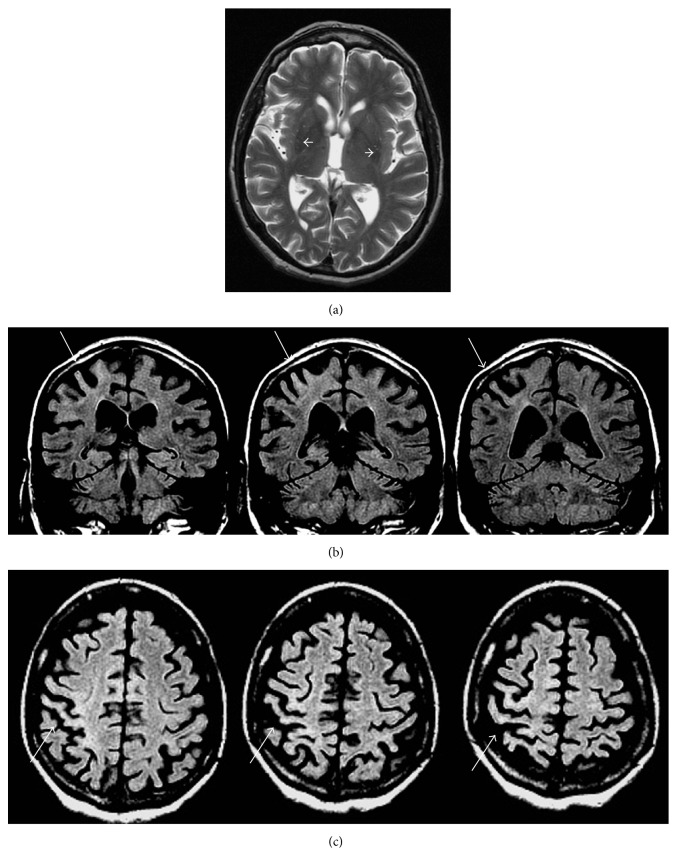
MRI changes disclosed in CBS. (a) Bilateral posterior putaminal T2-hypointensity (white arrows), mainly in the right side, where the putamen appears atrophic. (b)-(c): asymmetric cortical atrophy, mainly at the level of the right primary sensory-motor cortex associated with FLAIR hyperintensity (white arrows), on coronal (b) and axial images (c).
